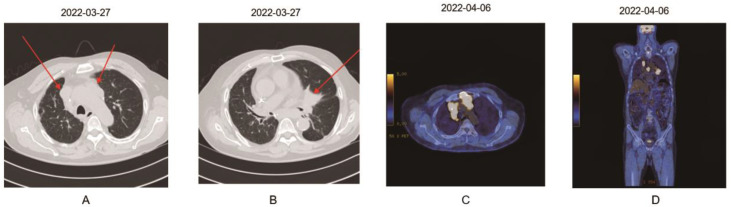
CT scan of the chest and [F-18]PET/CT scan on progression. (A,B) The CT scan of the chest on 22 March 2022 showed increased size of an increasing mass in central area of left upper lobe and enlarged LNs, as well as several new small nodules in both lungs. (C,D) The [F-18]PET/CT scan on 6 April 2022 showed [F-18]FDG uptake in metastatic nodules in the left and right upper lobes.
